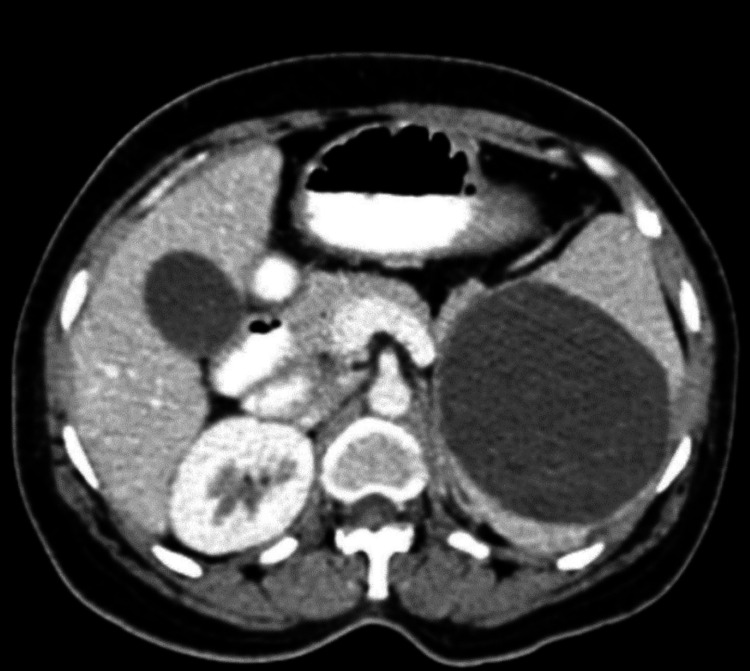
CECT image showing splenomegaly along with features of splenic hydatid cyst. CECT: contrast-enhanced computed tomography.
